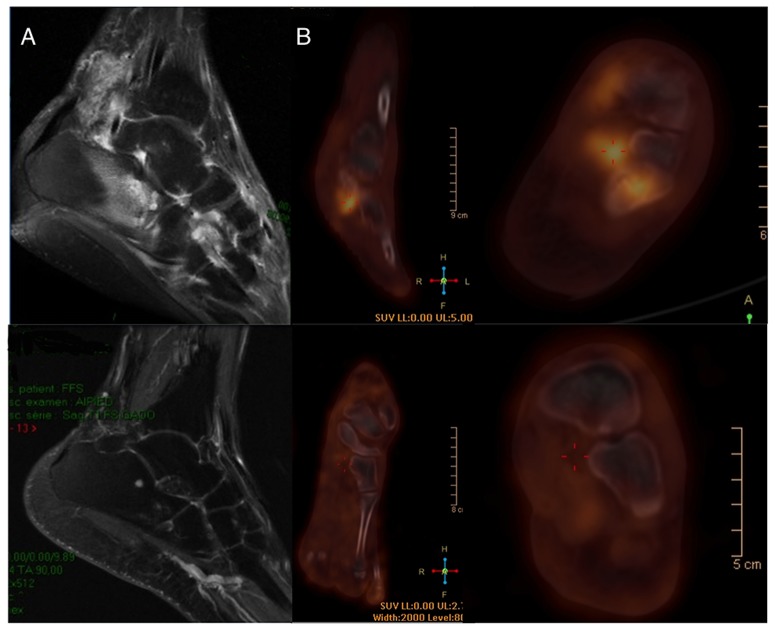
Major MRI and PET/CT responses in Patient 9. Left foot T1 Fat Sat Gadolinium enhanced MRI (A): Disappearance of periachillean tissular infiltration with the dot in the circle pattern, talus bone edema disappearance and slight talus contrast enhancement persistence. Disappearance of talus and tarsal hypermetabolism on left foot PET/CT (B).
